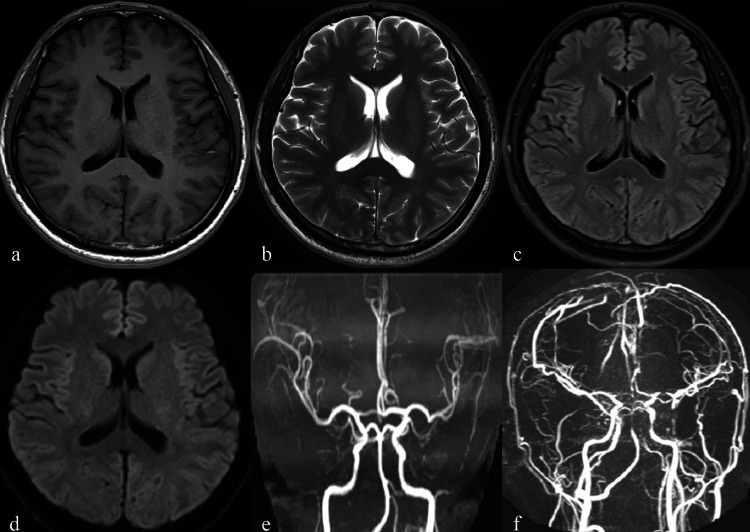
Images of the first onset. a-e: MRI and MRA showed unremarkable parenchymal and arterial structures. f: MRV demonstrated that the posterior part of the superior sagittal sinus, bilateral transverse sinuses, and sigmoid sinus were not visualized. The cerebral surface veins were significantly tortuous and thickened. MRI: magnetic resonance imaging; MRA: magnetic resonance angiography; MRV: magnetic resonance venography
